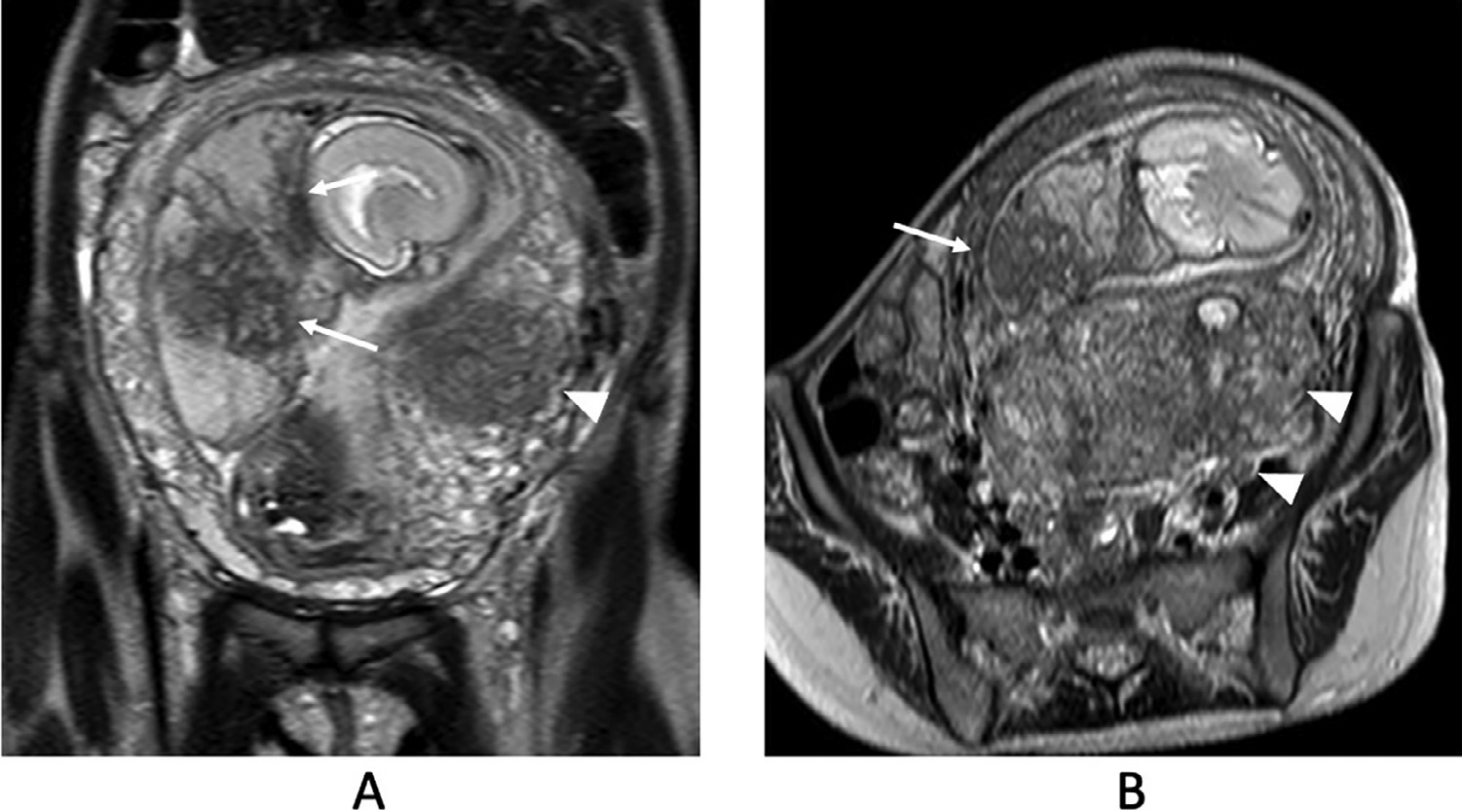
Single-shot turbo spin-echo images (T2-weighted images). (A and B) MRI obtained at 27 weeks of gestation during the previous pregnancy. The T2-weighted images obtained at 27 weeks of gestation at the previous pregnancy also demonstrate the same findings, such as intraplacental linear or geographical hypointensity (arrows) with oligohydramnios. Adenomyosis is also observed (A arrowheads).
